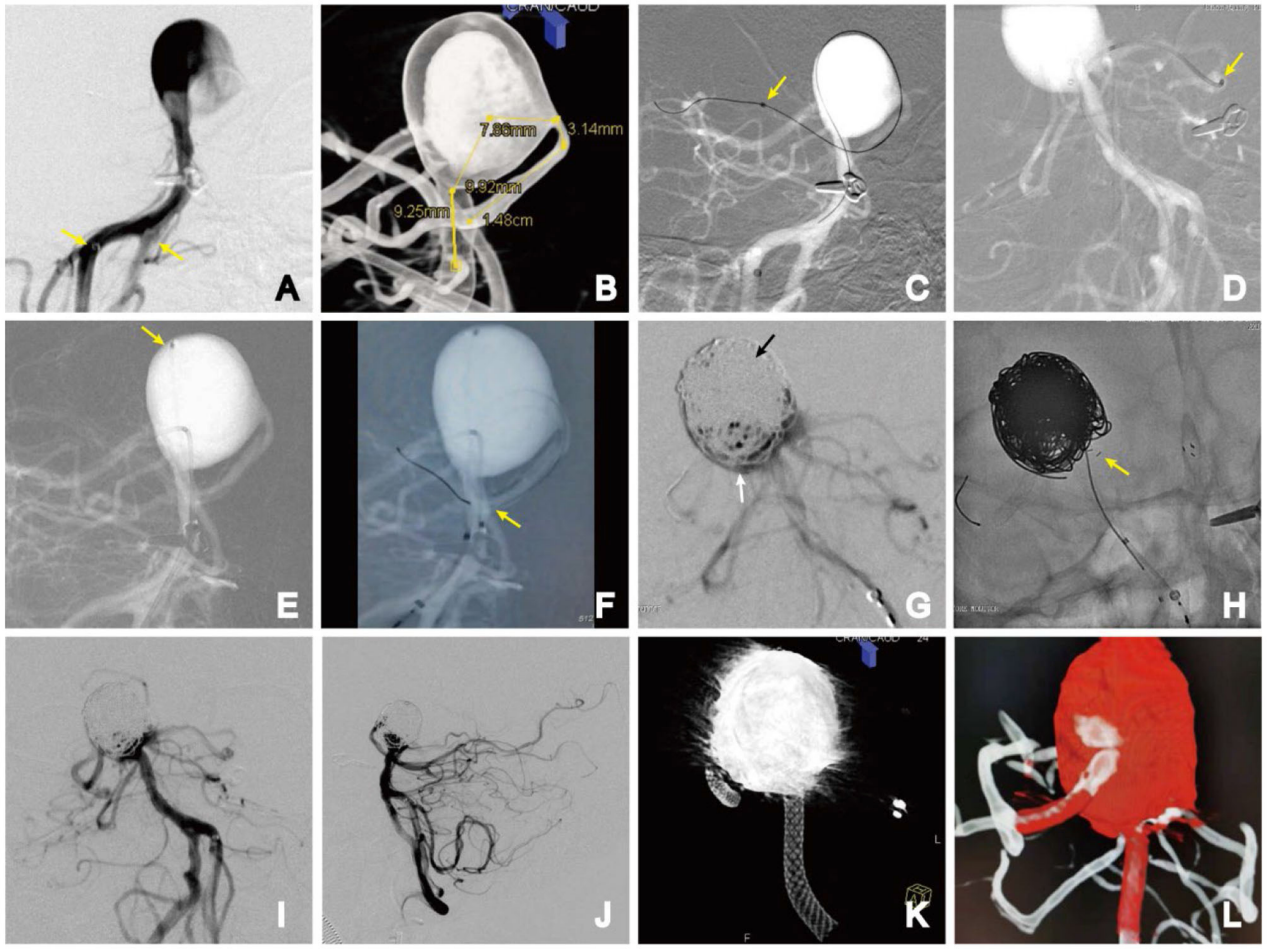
Stage 1. (A) Bilateral vertebral artery access (yellow arrows); (B) parent artery length, proximal and distal diameter, and aneurysm neck width were measured; (C) microguidewire-assisted microcatheter looping technique for super-select right posterior cerebral artery. The distal end of the Phenom catheter (yellow arrow); (D) distal end of the stent catheter in the left posterior cerebral artery (yellow arrow); (E) position of the coiled catheter (yellow arrow); (F) PED semi-deploying in the middle segment of the basilar artery. The anchor point of the distal end of the PED (yellow arrow); (G) densely packed upper part (black arrow) and slack coiled lower part (white arrow) of the aneurysm; (H) implanted E2 stent, achieving full expansion in front of the aneurysm neck in a T-fashion. AP (I) and lateral (J) DSA images; (K) Dyna-CT volume imaging showing the PED was fully deployed; (L) dual-volume reconstruction imaging showing all the vessels were patent.
